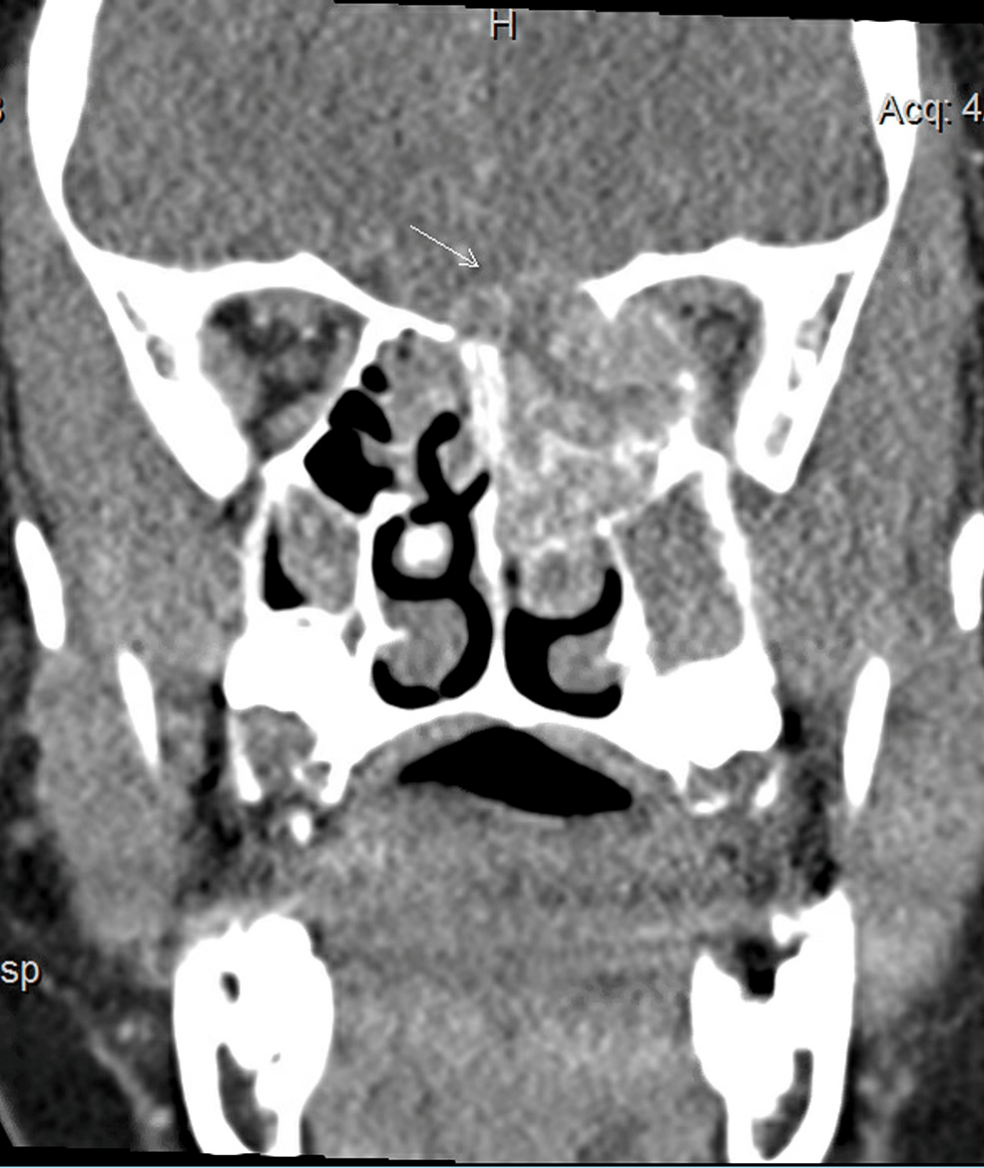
Maxillofacial CT scan of face and neck with contrast The image demonstrates significant diffuse sinus opacification (left greater than right) with areas of osseous dehiscence along the left medial orbital wall and extension into the left upper nasal space. Superiorly, there is erosion of the floor of the anterior cranial fossa with extension of the ethmoid air cell opacification into the left and right frontal lobes (arrow) CT: computed tomography
